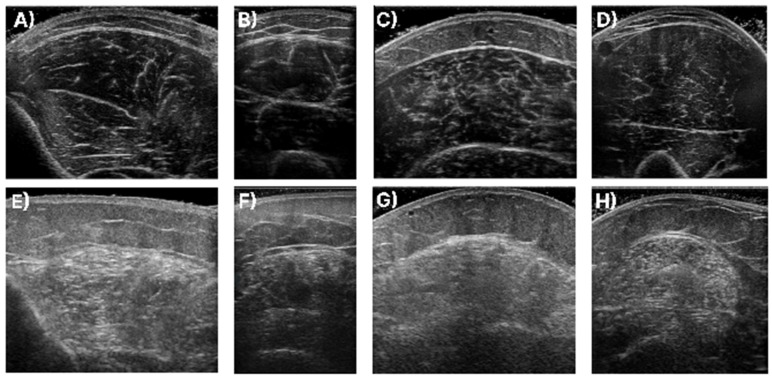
Sample of ultrasound recordings with normal and high echogenicity. (A,E) shows images from the T.A., (B,F) shows images from the R.F., (C,G) shows images extracted from the GCM, and finally, (D,H) are images of the B.B.
